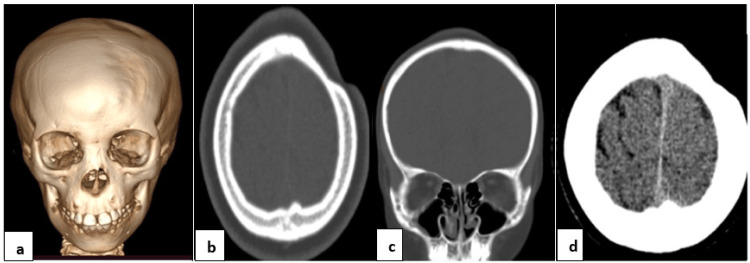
Head CT scan 3D volume rendering (a), axial bone window (b), coronal bone window (c), and axial brain window (d) showing thinning of the left frontal and parietal bones, subcutaneous tissues/fat, and reduction in the left nasal cavity. Subtle effacement of the sulci of the left frontal lobe is also seen. CT, computed tomography; 3D, three-dimensional.
